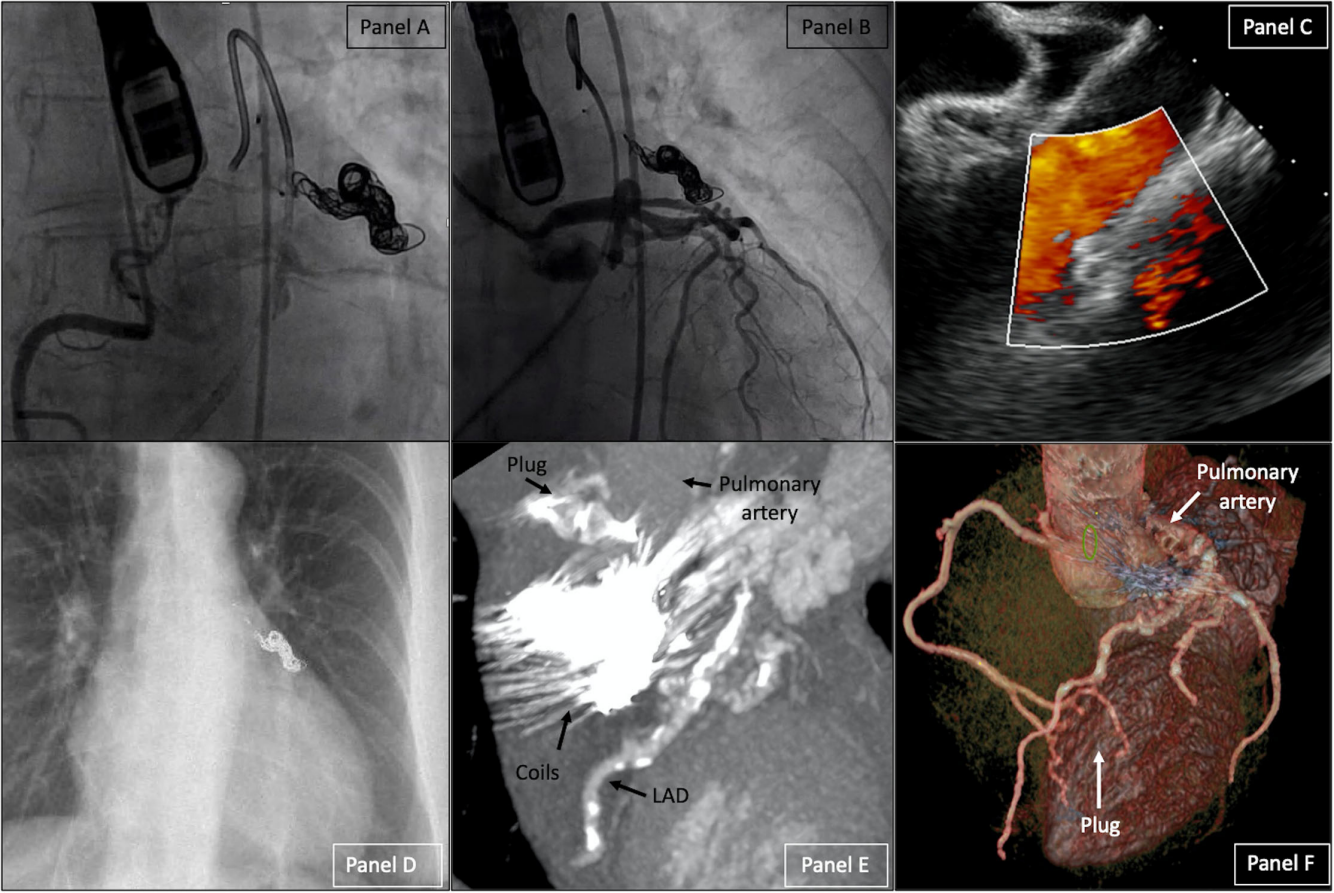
Right Coronary Artery (RCA) angiogram (A), Left Coronary Artery (LCA) angiogram (B), TOE (C), chest radiography (D) after fistula closure. CCT after the intervention with 2D Maximum intensity projection of the fistula (E) and 3D volume rendering of the heart (F). TOE, *transesophageal echocardiogram;* CCT, *cardiac CT*.
